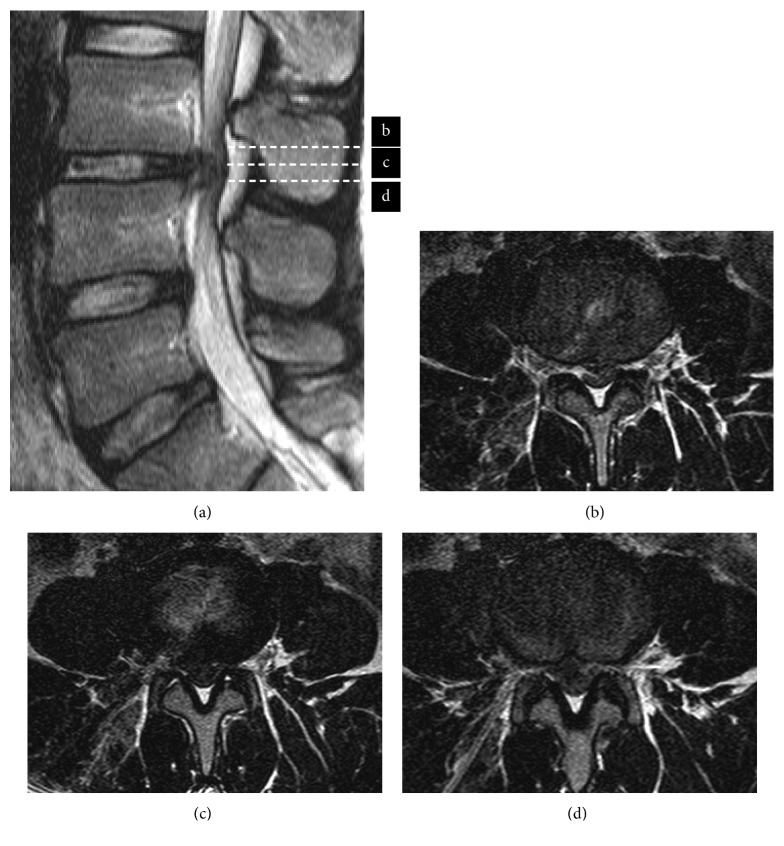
The first postoperative T2-weighted MRI did not change disc herniation at L3/4 disc level (a), and trajectory did not reach disc herniation (b–d).
